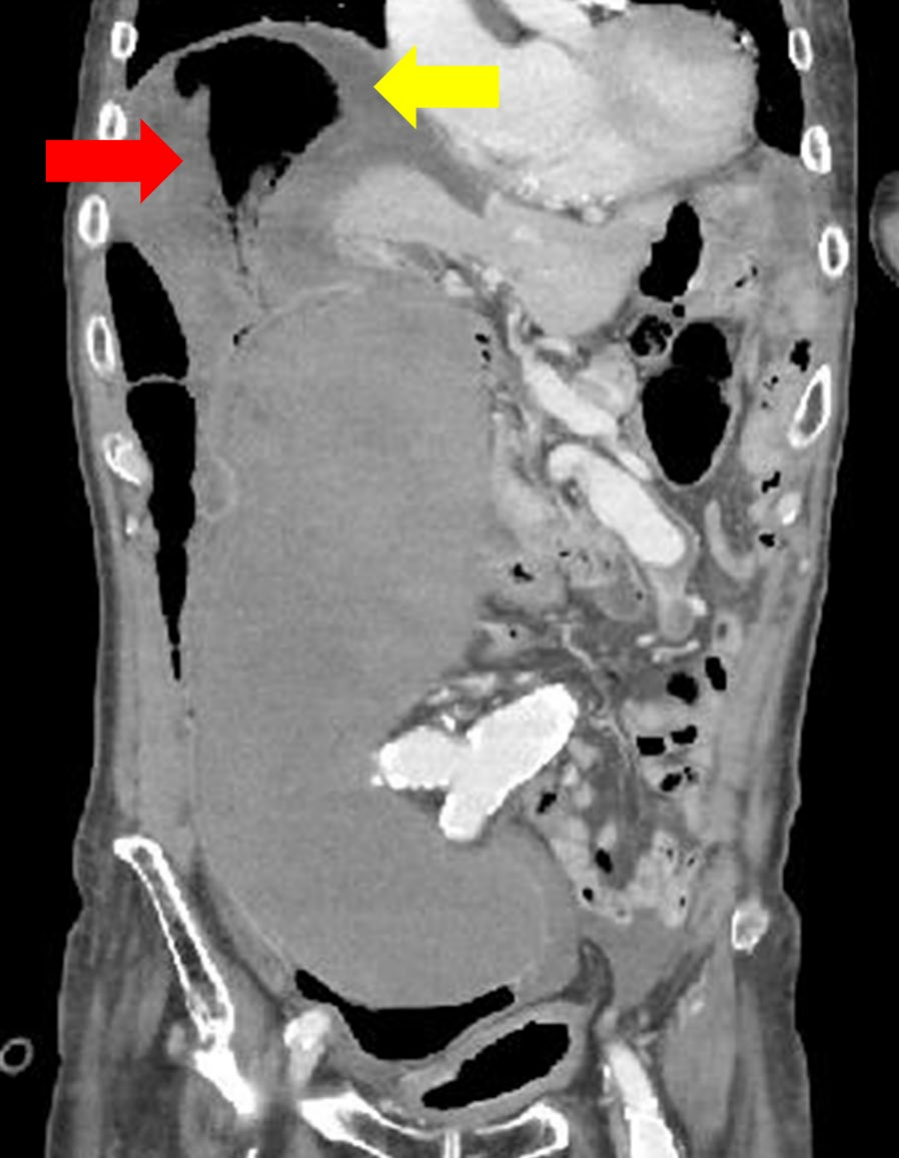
Abdominal contrast-enhanced CT scan on the first day in the hospital (equilibrium phase). The contrast effect on the sigmoid colon wall, compressed by the hematoma, is poor (red arrow) and the ascites are increased (yellow arrows). CT: computed tomography
